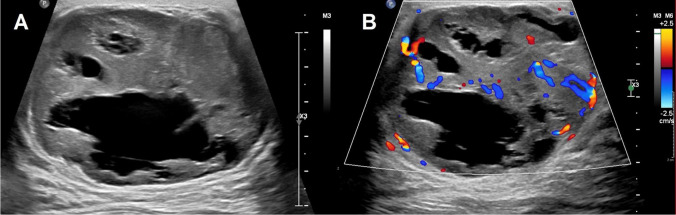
Breast ultrasound with indication of a palpable lump in a 17-year-old female showed a complex cystic and solid mass with circumscribed margins with internal vascularity in the left breast at 7:00 position, 5 cm from the nipple. Ultrasound image (A) shows a complex cystic and solid mass with circumscribed margins at the 7:00 position, 5 cm from the nipple, measuring 6.2 × 5.3 × 6.7 cm with internal vascularity (B). This finding was recommended for further evaluation at the breast clinic by the pediatric radiologist. Finding was given a BI-RADS 4 assessment by the breast imaging radiologist at the follow-up examination and subsequently biopsied resulting in malignant spindle cell tumor
